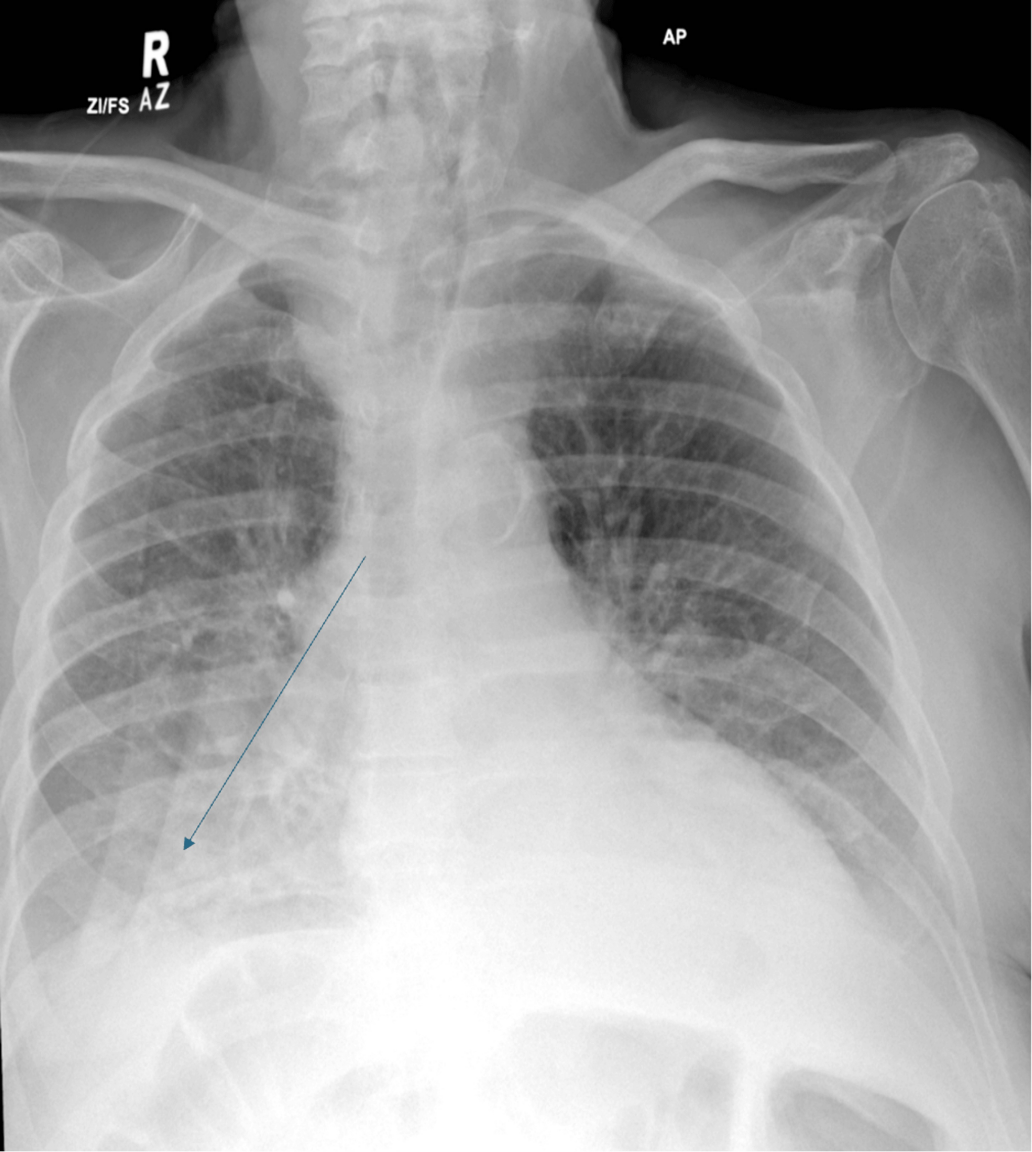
Chest X-ray taken on 24 October 2024 showing right-sided haziness and blunting of the costophrenic angle, suggestive of pneumonia. The patient was subsequently started on antibiotics on October 26, 2024.
